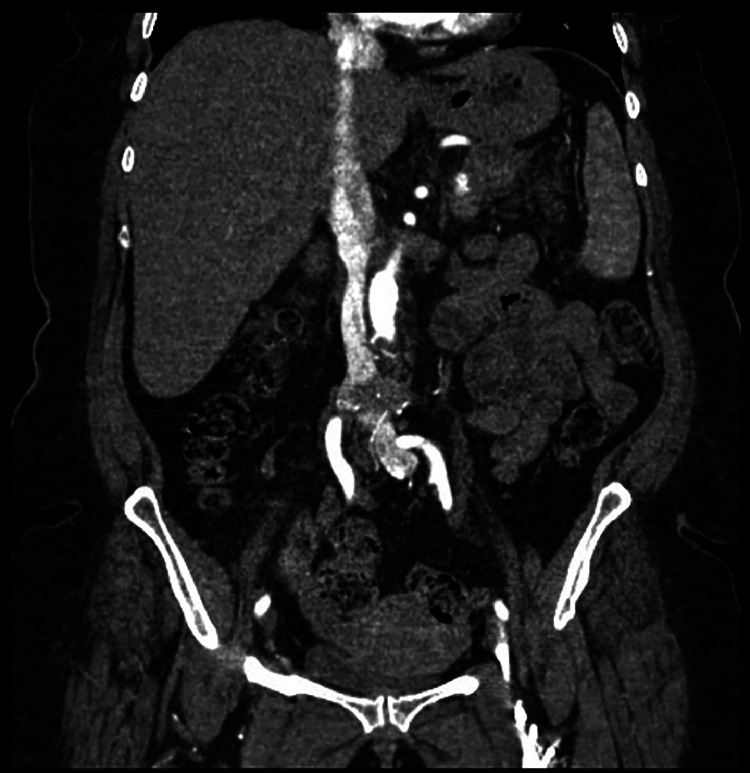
A coronal CT image obtained after open surgical removal of the IVC filter. The aorta appears intact with no visible filter struts or wall abnormalities. No residual thrombosis is observed at the prior site of strut penetration, indicating a successful repair (red arrow).
